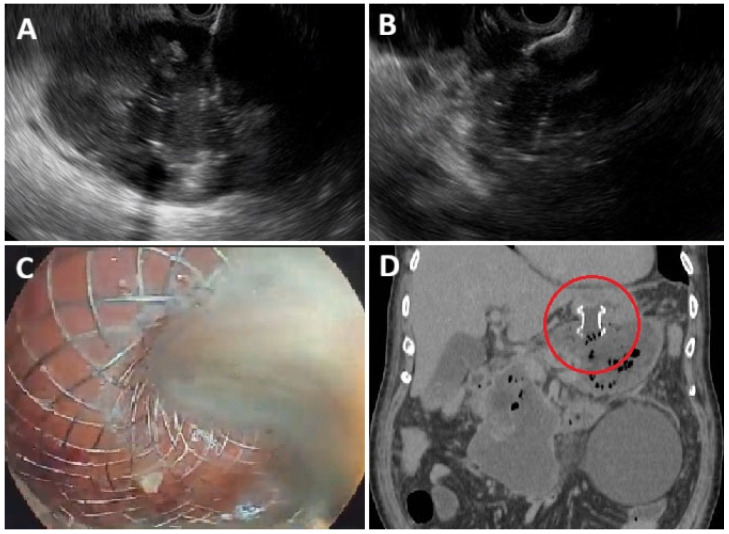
Procedural steps of EUS-guided drainage of a large walled-off necrosis (WON). (A) EUS view showing the WON. (B) Deployment of a lumen-apposing metal stent (LAMS) within the WON. (C) Drainage of purulent material through the LAMS. (D) Abdominal CT scan demonstrating a transgastric LAMS in place (red circle) for WON drainage.
